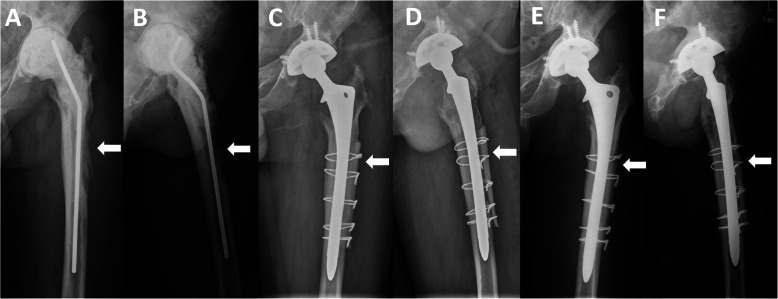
Radiographs of a 44-year-old man who underwent revision THA for periprosthetic joint infection. a, b Anteroposterior and lateral radiographs prior to stage 2 revision THA showing a Paprosky type IIIA femoral bone defect. c, d Radiographs immediately after revision THA using an extensively porous-coated stem with cortical strut allografts, showing that the cortical strut allografts bridged the bone defects (white arrow) and supported the thinning cortex. e, f Postoperative radiographs at 9 years showing successful incorporation of the cortical strut allografts to the host bone, bone restoration in the bone defect area (white arrow), and a significant increase in femoral width. No sign of stress shielding and distal cortical hypertrophy was observed. The stem was assessed as bone ingrowth stable
